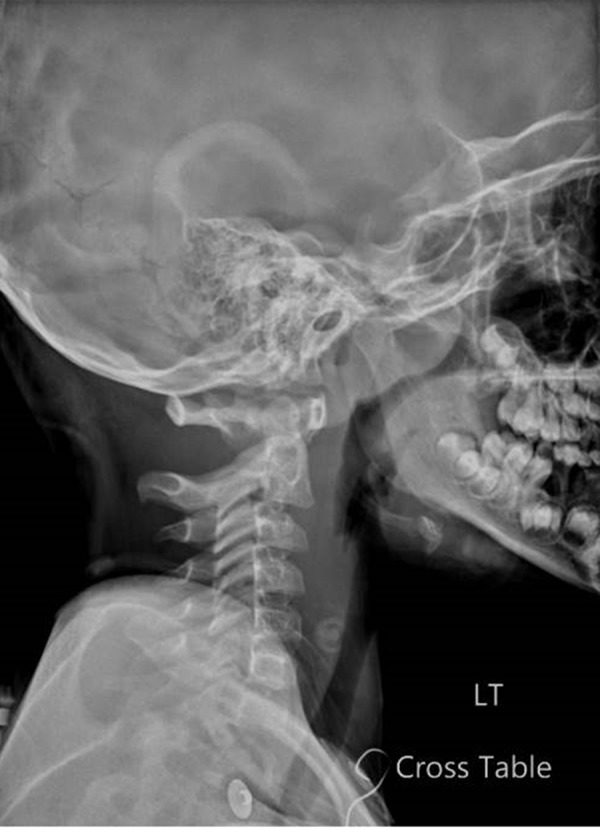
Lateral X-ray of the cervical spine revealing mild anterolisthesis of C2 on C3.
